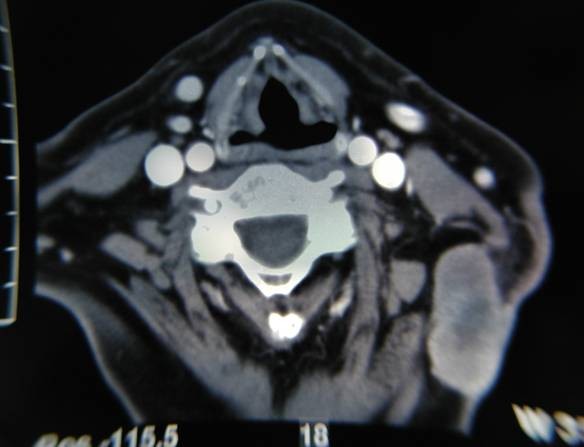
Axial CT scan with contrast of the neck showing the lesion with peripheral enhancement and hypodense necrotic center in the subcutaneous tissue of the posterior neck triangle.
